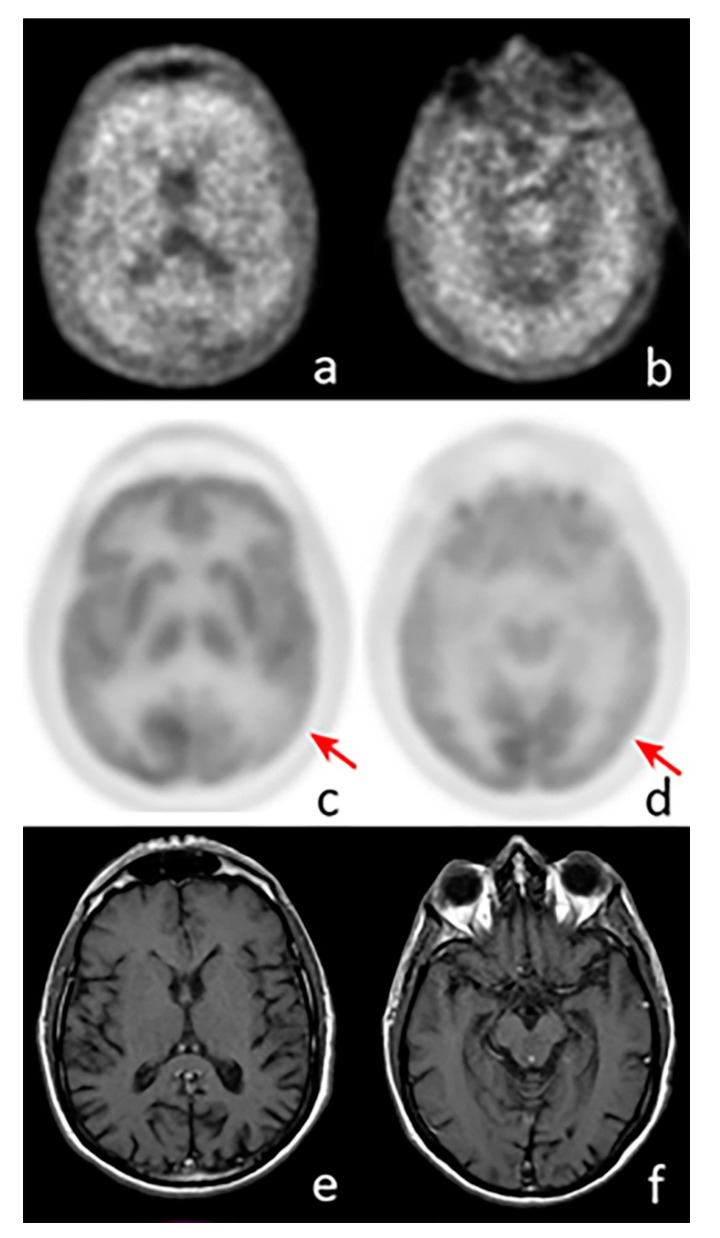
Axial images of a positron emission tomography (PET) scan performed with ^18^F-Florbetaben (a,b), ^18^F-Fluorodeoxyglucose (c,d), and MRI (e,f) in a patient with suspected AD. The images show pathological amyloid burden in the cortex, while a significant reduction in cortical glucose consumption is visible in the left parietal (c, arrow) and temporal lobes (d, arrow). MRI does not show any abnormal findings.
